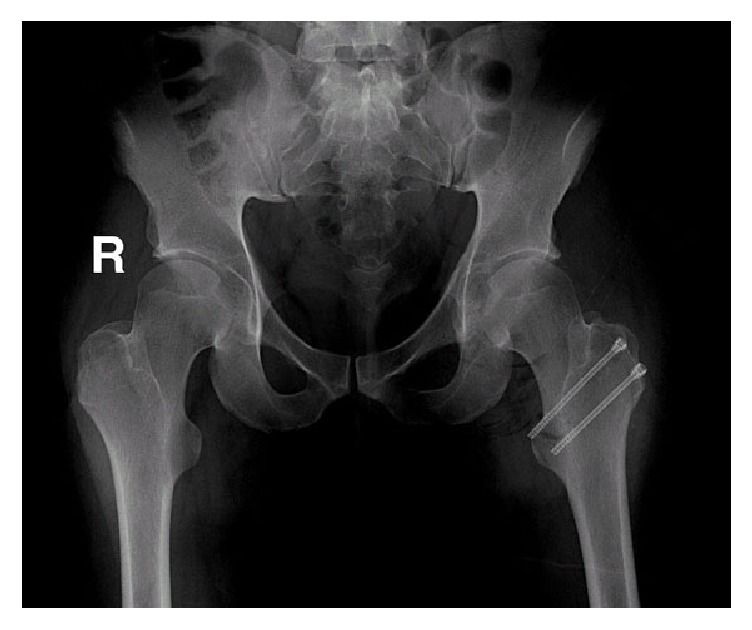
Postoperative radiographs showing the greater trochanter reattached using 2 cannulated 6.5 mm screws.
